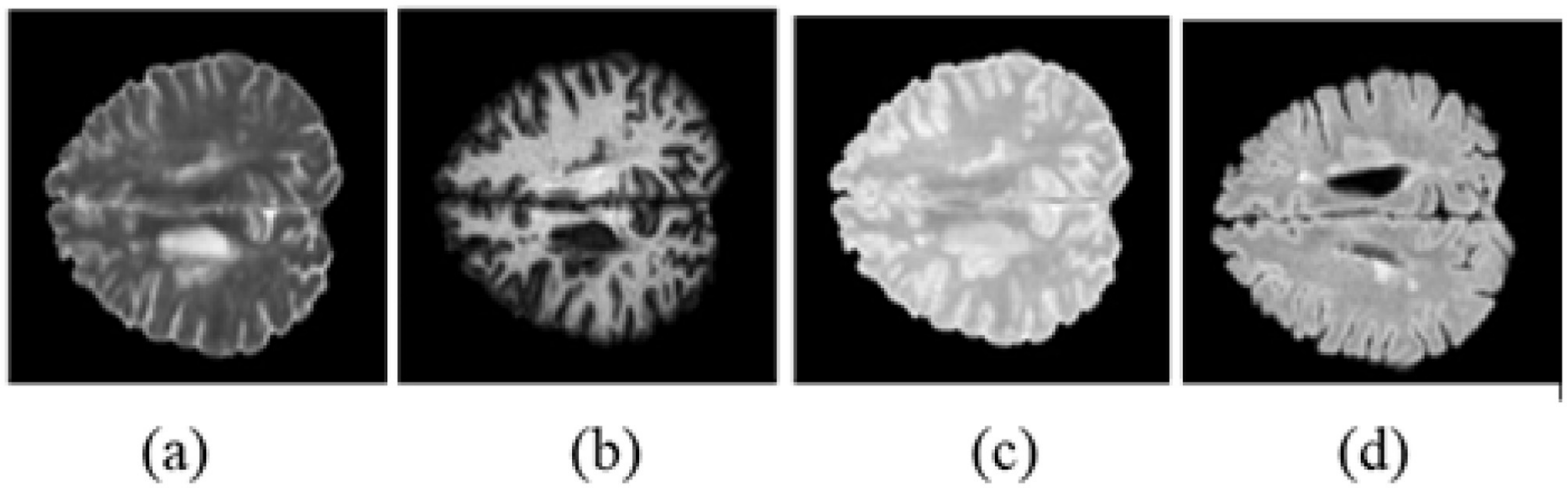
ISBI-2015 sample brain MRI images in different image modalities when slice number is 100 for the first patient. (a) T2-weighted, (b) MPRAGE, (c) PD, (d) FLAIR.
